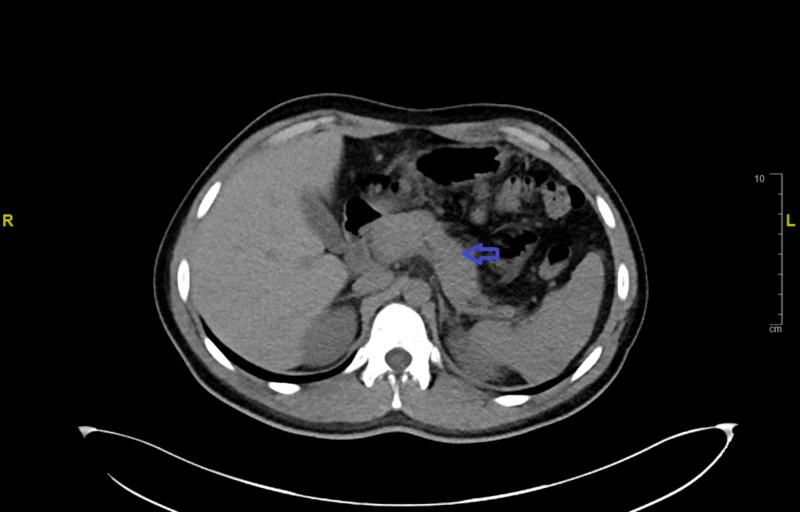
CT of the abdomen without intravenous or oral contrast showed acute pancreatitis (blue arrow)
